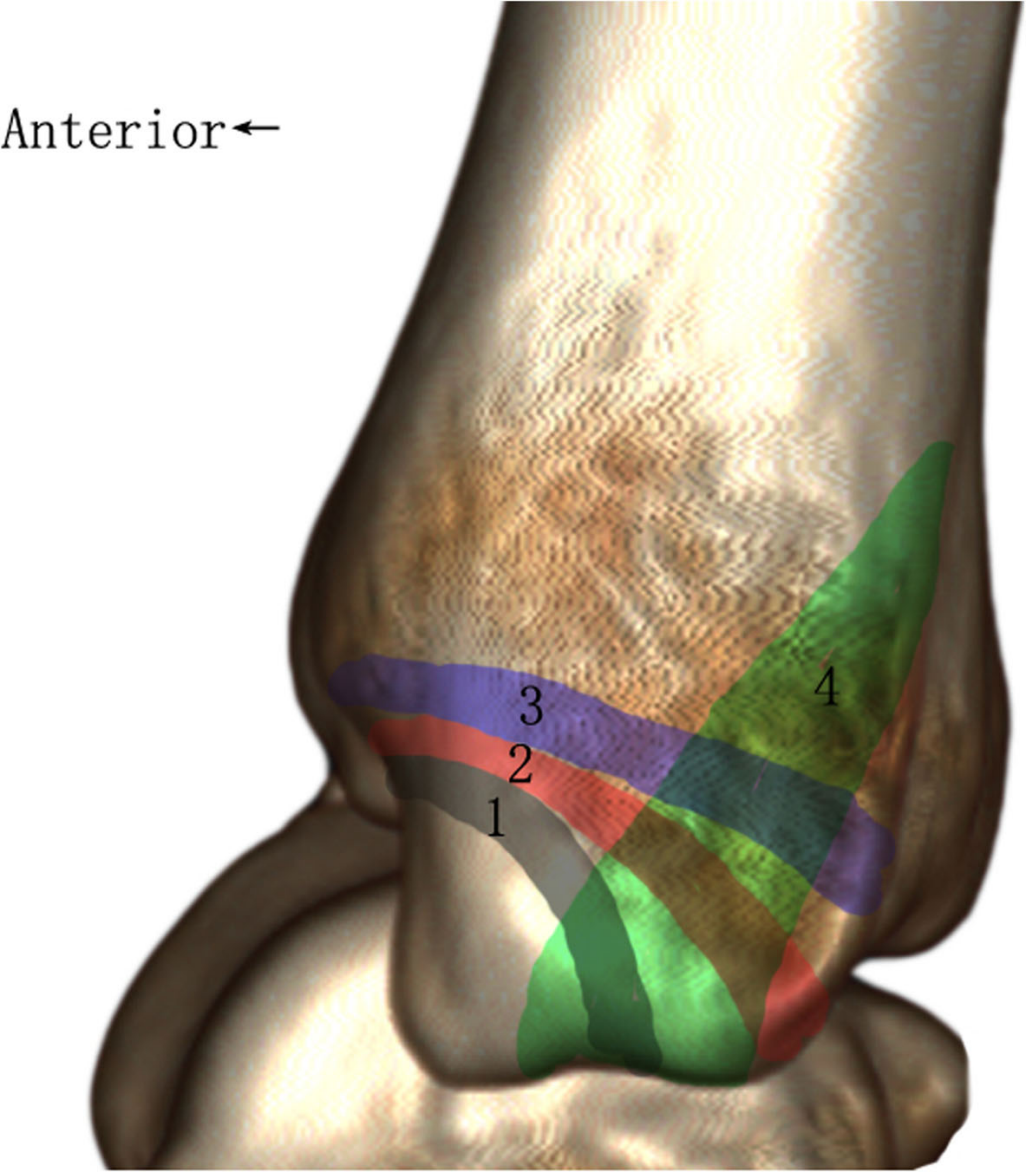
The new fracture line system to classify the medial malleolar fractures according to the 3-D reconstruction CT
